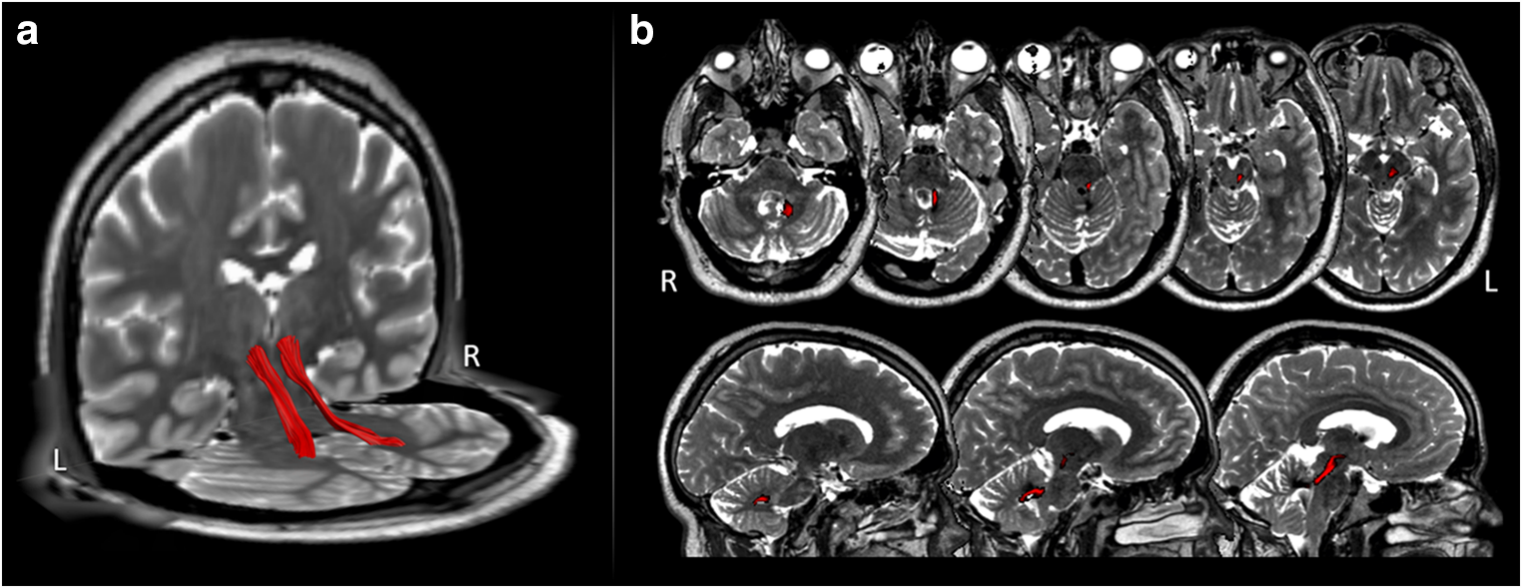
Dentatorubrothalamic tract dissection: 3D (a) and 2D (b) dissection of the dentatorubrothalamic tracts (red) imposed on the patient’s T2 volumetric MRI
